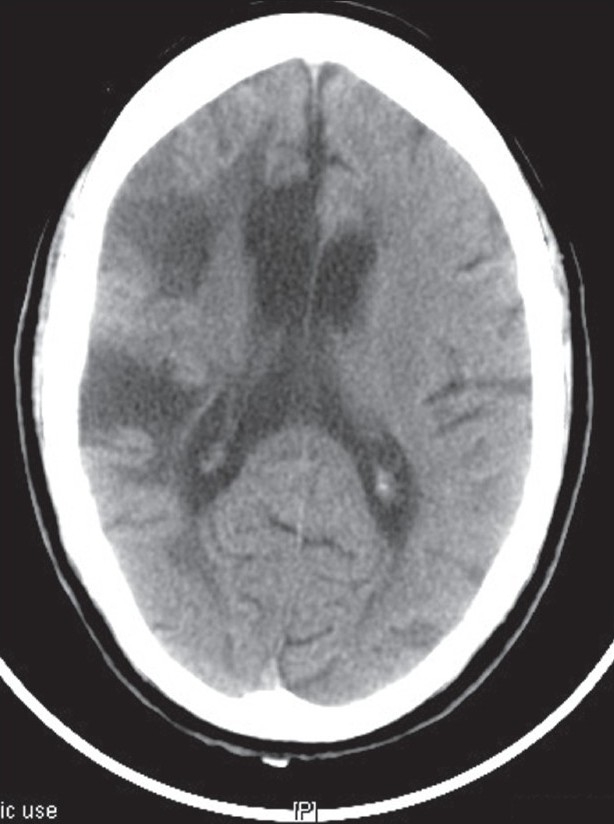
CT head revealed a wedge shaped acute infarct in right middle cerebral artery territory along with areas of enchephalomalacia and gliosis in right fronto-temporal and temporo-parietal lobe and in paraventricular white matter with ex-vacuo-dilation of frontal horn of lateral ventricle, suggestive of chronic infarct of middle cerebral artery territory
